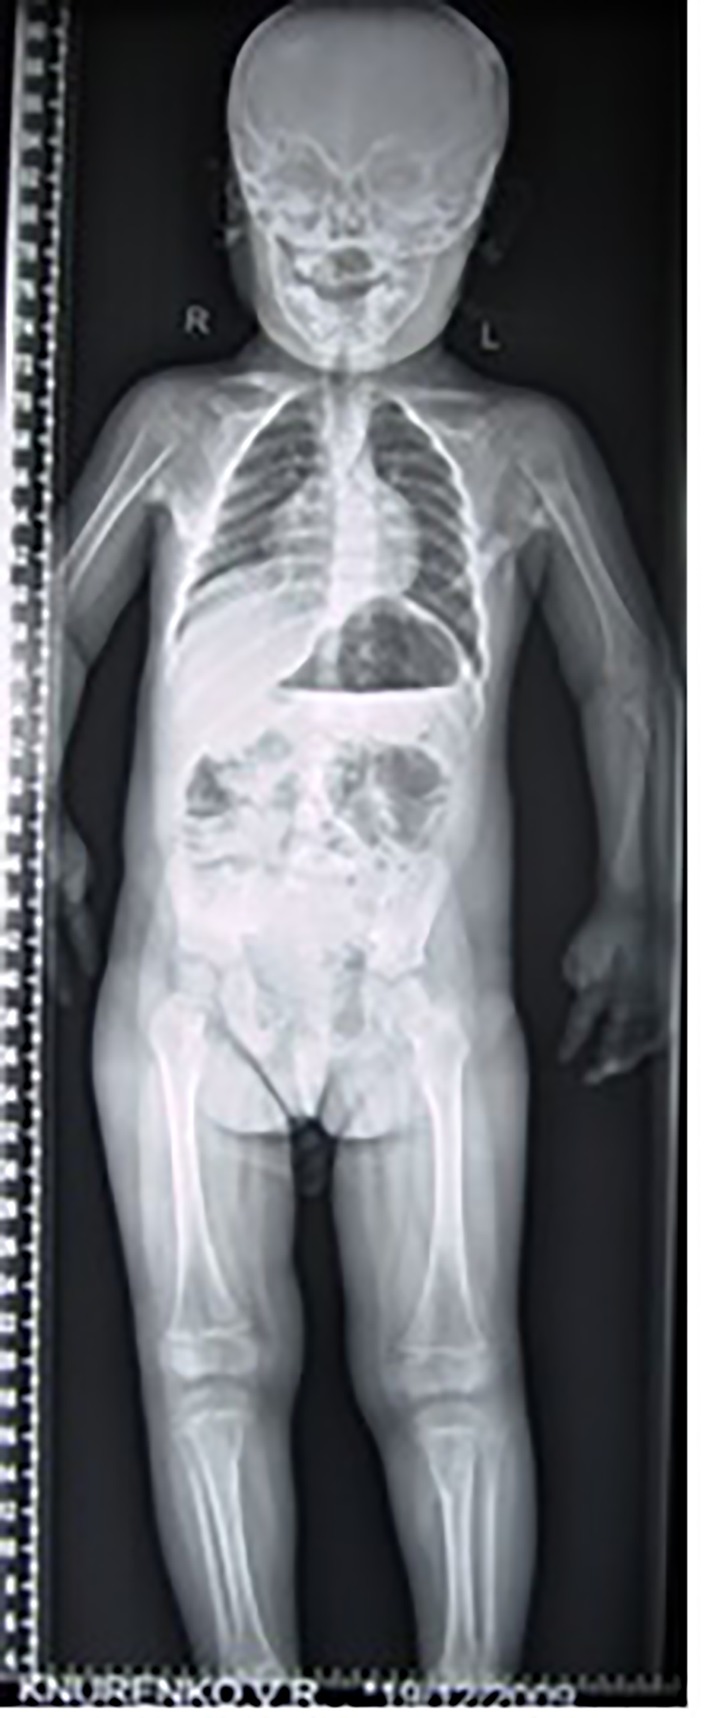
AP thorax radiograph showed the columns and plaques of ectopic bone in a 7-year-old girl with FOP and situs inversus (note the direction of the apex of the heart). Opacities in the muscles and near the tendons insertions were encountered in all patients with progression from proximal to distal and formation of a true bony bridge between various parts of the skeleton was evident (arrows).
